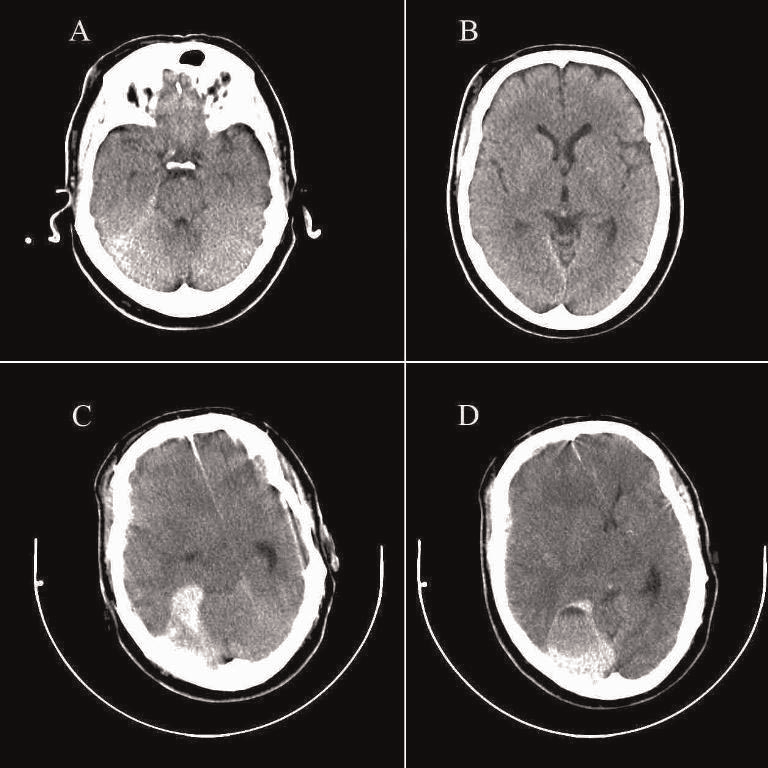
Non contrast enhanced CT scan at initial presentation on the emergency room (A and B) and 12 hours after admission (C and D).
